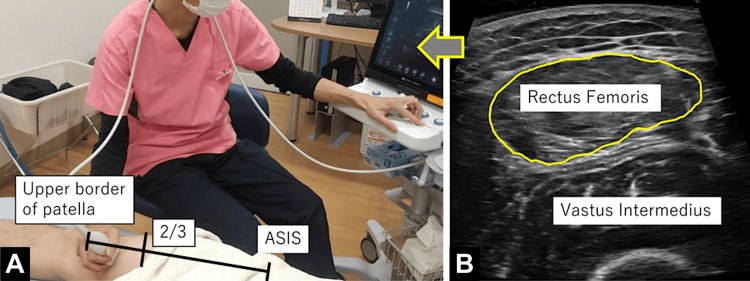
Ultrasound image and examination scene of the rectus femoris muscle. (A) Examination setup. The anatomical measurement point is marked at approximately two-thirds the distance from the anterior superior iliac spine (ASIS) to the upper border of the patella. (B) Ultrasound image. Cross-sectional view of the rectus femoris and vastus intermedius muscles, with the rectus femoris area outlined in yellow. Original image by the authors.
